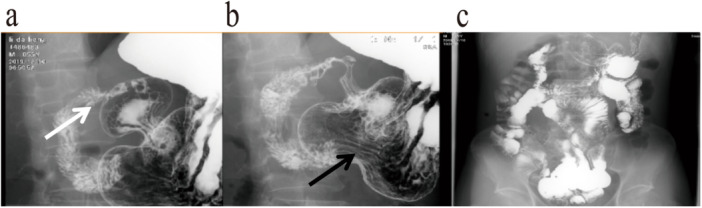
Alimentary tract contrast imaging in Case 1: Dual involvement of duodenum and ileum. Contrast radiography reveals two distinct abnormalities: (a) duodenal ulceration and stricture in the duodenal bulb (white arrow), corresponding to the endoscopic findings shown in Figure 1; (b) ileal stenosis in the distal ileum (black arrow); and (c) multiple intestinal lesions in the duodenum and distal ileum.
